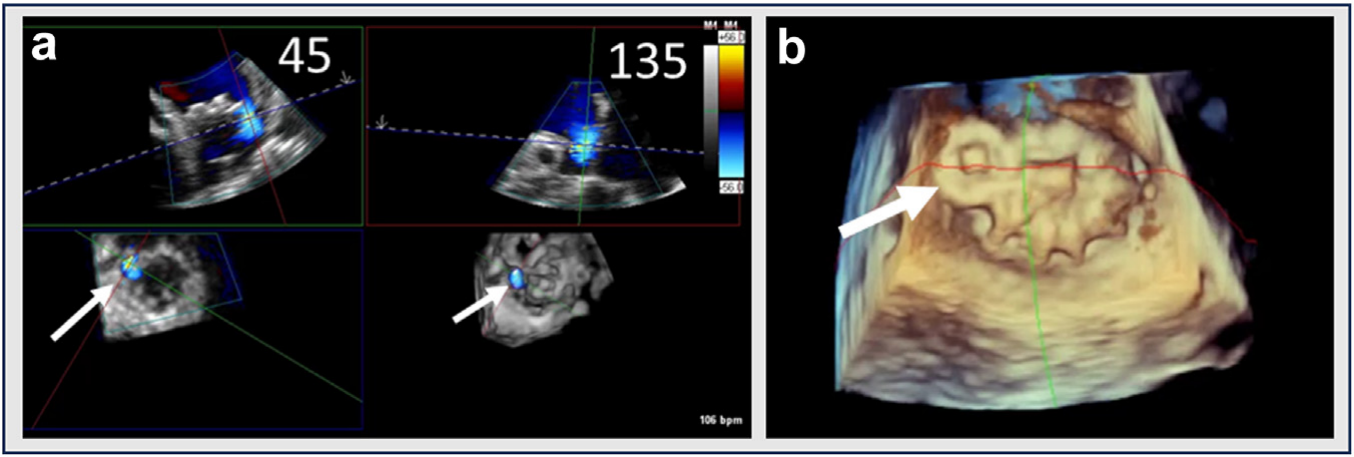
PDL closure with an AVP 2 device. (a) Significant PDL was identified by TEE (similar to the patient in Figure 3). (b) The PDL was wired and closed with a single 12 mm AVP 2 device (arrow), which is deliverable through a standard 5F catheter Abbreviations: AVP, amplatzer vascular plug; PDL, peridevice leak; TEE, transesophageal echocardiography.
